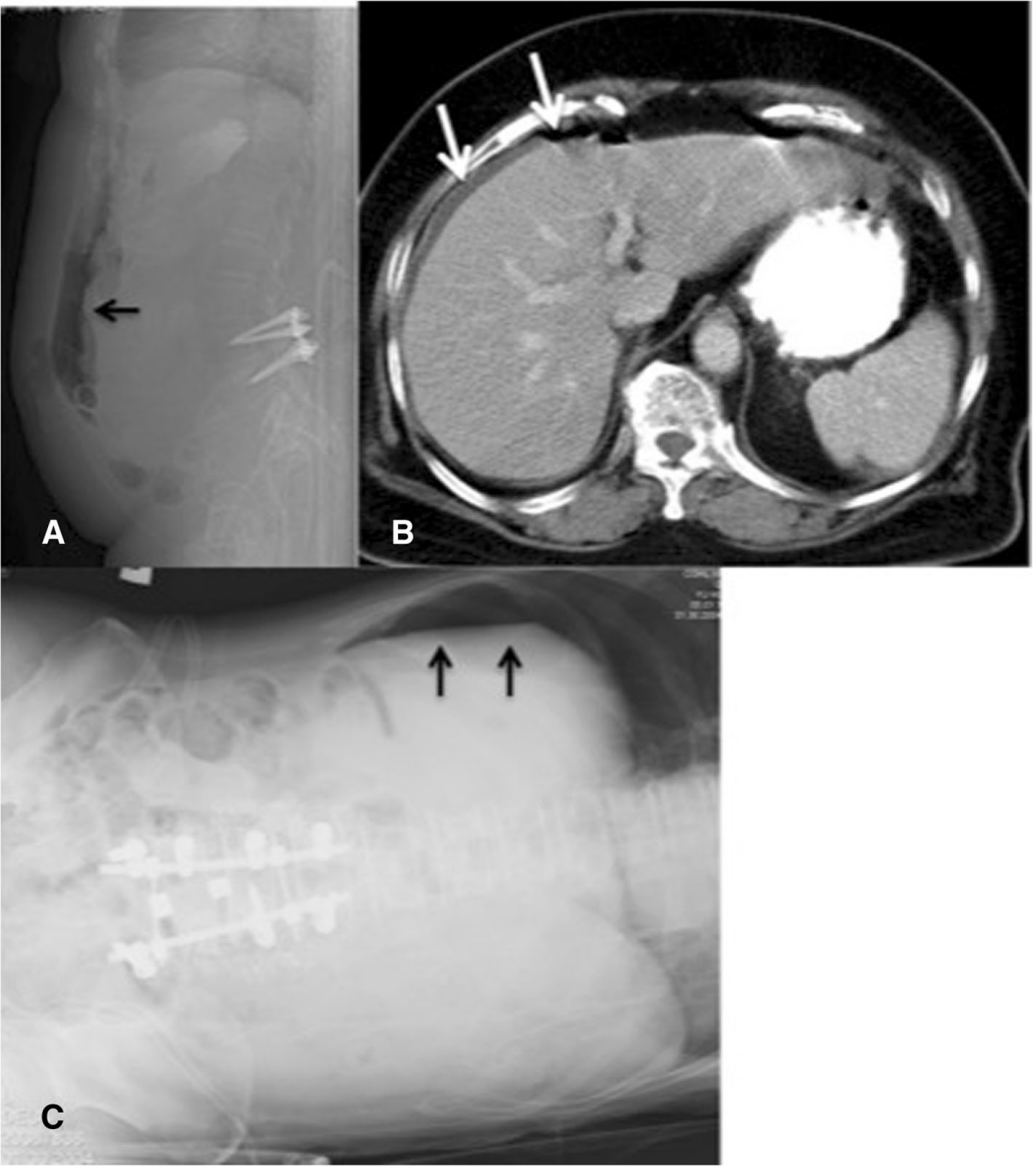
A, B A 76-year-old female complained of abrupt onset of abdominal pain with progressive muscle guarding, which started on postoperative day 1 after lumbar decompression and posterior instrumentation at L4–5. A Supine lumbar lateral radiograph showed intra-abdominal free air without disruption upon examining the bowel gas pattern (black arrow). B Axial computed tomography (CT) revealed intraperitoneal free air and fluid in the right paracolic gutter (white arrow). C A 70-year-old male experienced sudden abdominal pain with abdomen distension, which started on postoperative day 3 after lumbar decompression and posterior instrumentation of L3-S1 Intra-abdominal free air and an air-fluid level were observed on the left lateral decubitus view (black arrow)
